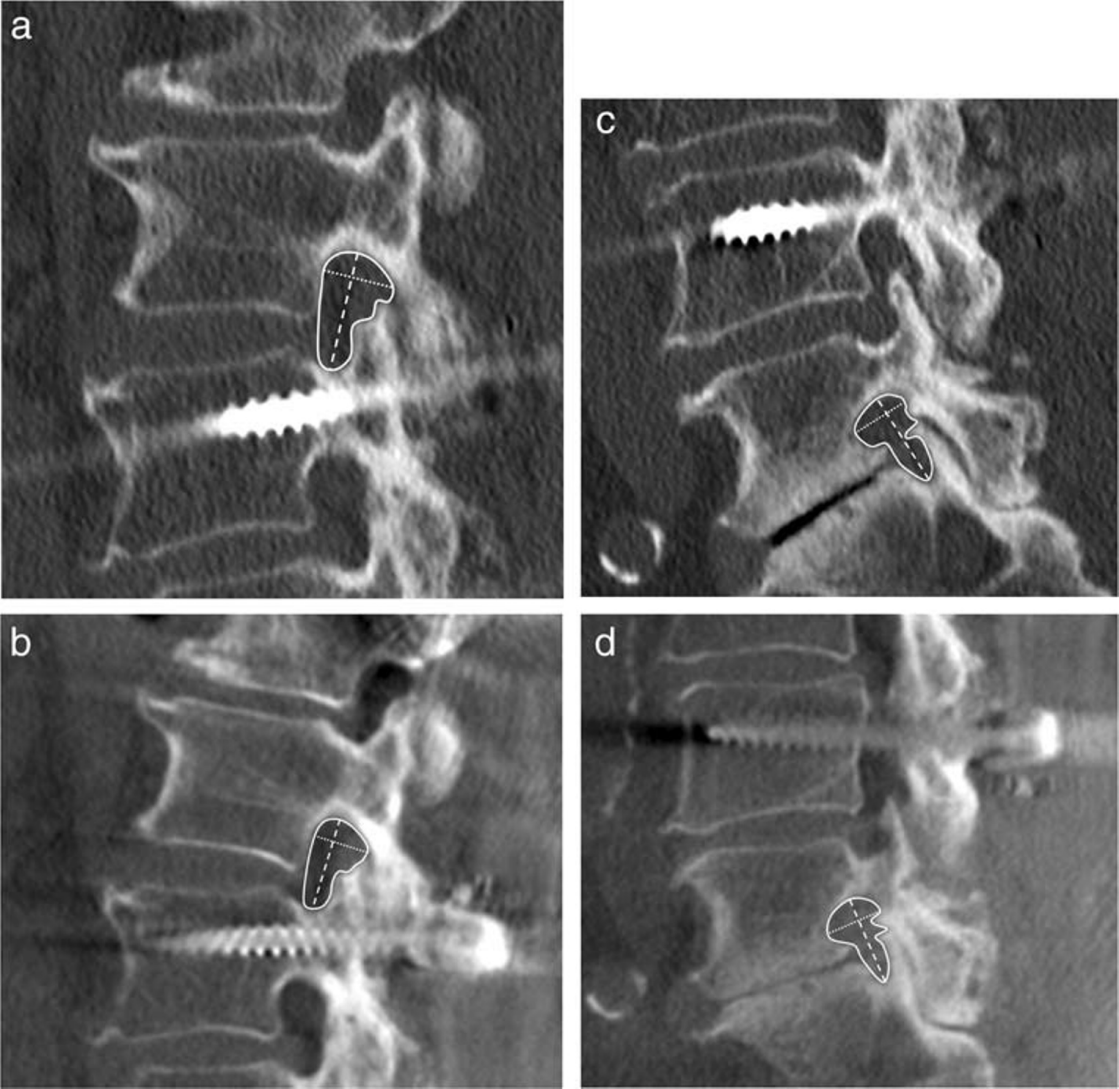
Comparison of the right L2 and L5 neural foramen in a 68-year-old female on supine CT (a/c) and upright 3D tomography (b/d). L2 neural foramen with normal intervertebral disc height shows a decrease in size from 1.29 cm^2^ for cross-sectional area (continuous line), 1.8 cm for cranio-caudal (dashed line), and 1.1 cm for ventro-dorsal (dotted line) diameters in the non-weight-bearing position (a) to 1.20 cm^2^, 1.7 cm cranio-caudal, and 1.0 cm ventro-dorsal in the weight-bearing position (b), respectively. The size of the L5 neural foramen is unchanged for cranio-caudal (1.69 cm) and ventro-dorsal diameters (1.0 cm) but shows a slight reduction of the cross-sectional area from 1.04 cm^2^ in the non-weight-bearing to 0.94 cm^2^ in the weight-bearing position (c, d) due to preexisting severe osteochondrosis
